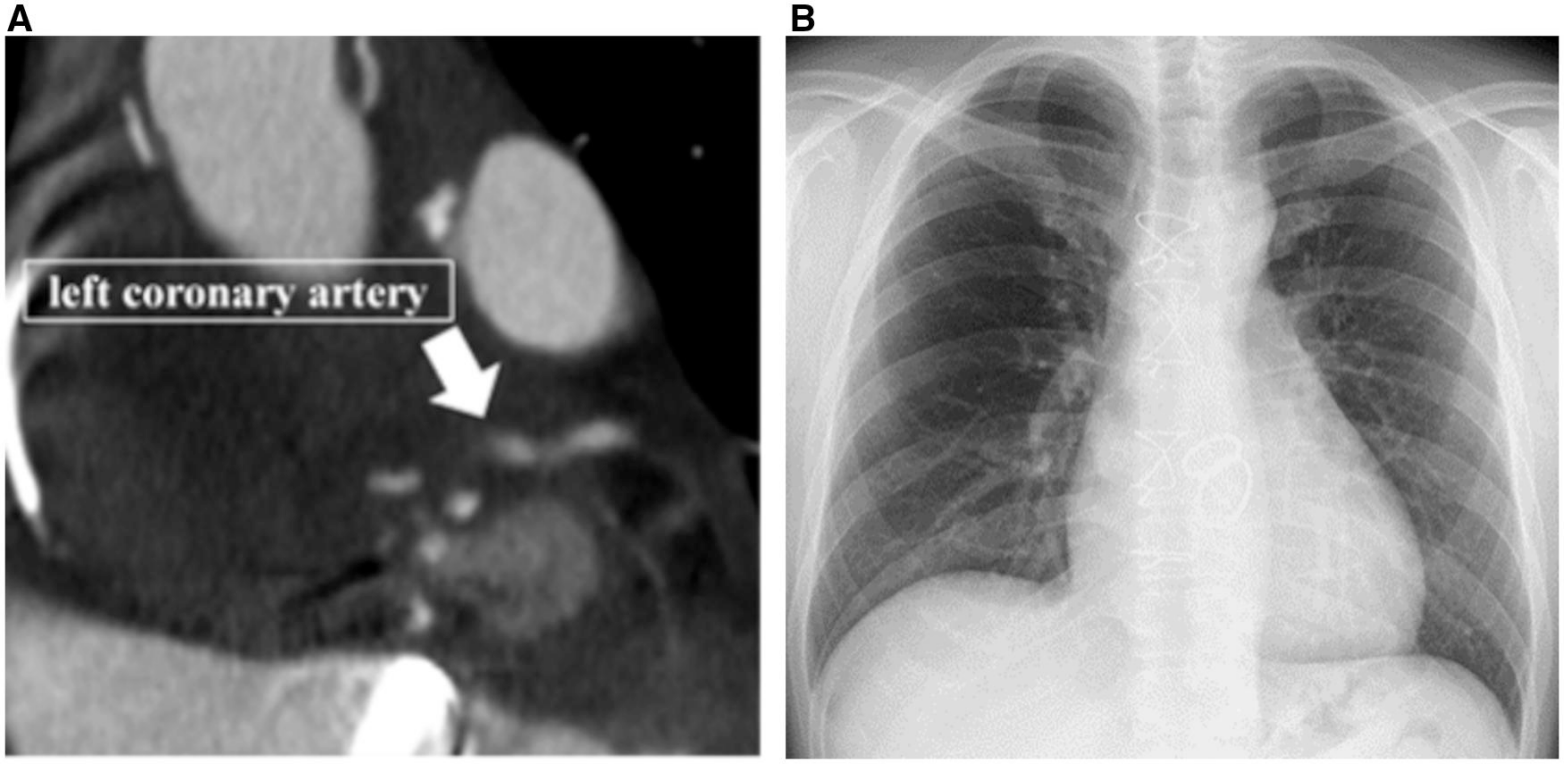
(A) Pseudoaneurysm under the left coronary button. (B) Postoperative chest X-ray.
